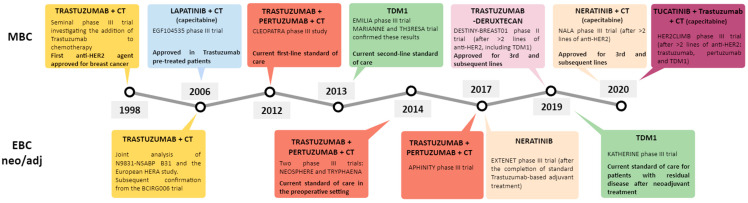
The evolution of human epidermal growth factor receptor 2 (HER2) blockade strategies in HER2+ breast cancer. CT = chemotherapy, EBC = early breast cancer, HER2 = human epidermal growth factor receptor 2, MBC = metastatic breast cancer, neo/adj = (neo) adjuvant, and TDM1 = trastuzumab emtansine.
